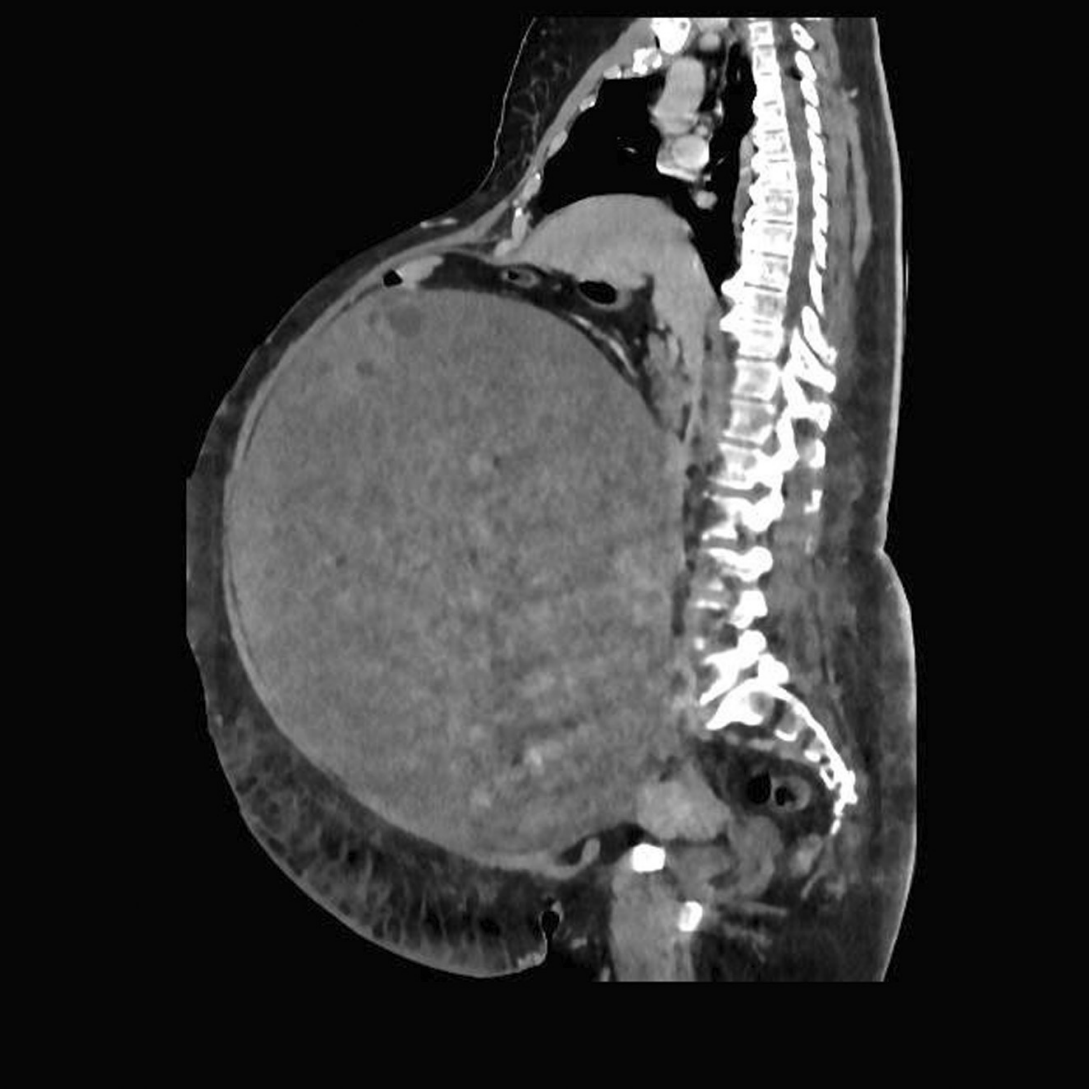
CT scan. Coronal view of the abdominal CT scan.
